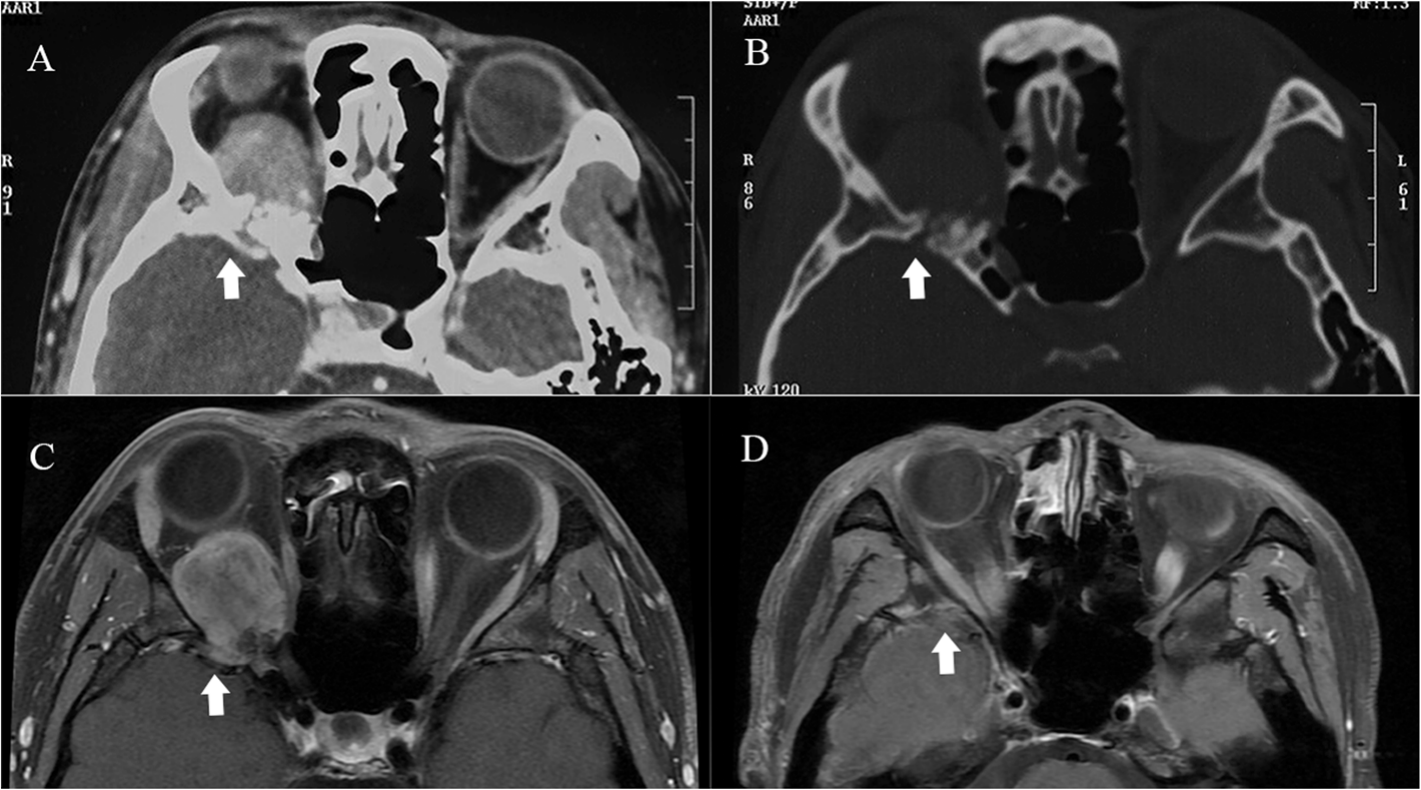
Case 4. The patient was a 25-year-old with chronic disease process and right exophthalmos for more than 6 months. a, b: CT showed that the tumor involved the bone of the lateral orbital wall with bone destruction (arrow). c: MRI showed that the main body of the tumor was located lateral orbit and invaded the intracranial dura through the superior orbital fissure (arrow). d: After the Transfronto-orbital approach was given to the craniotomy, the MRI re-examinations immediately after the operation showed that the tumors were removed completely. There was no recurrence after 7 years of MRI follow-up
